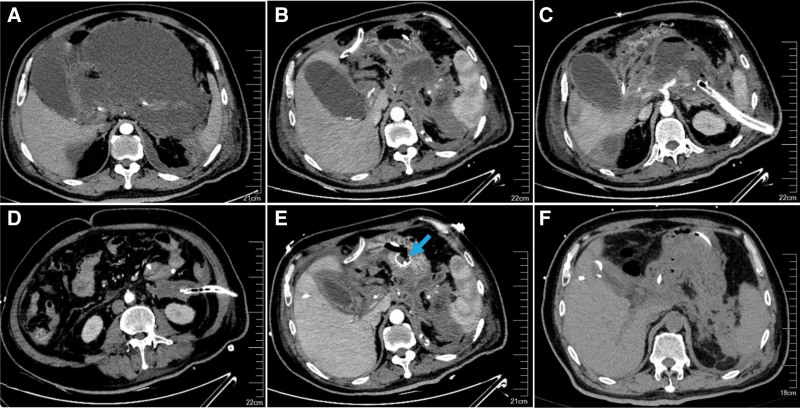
CT images of the abdomen in patient 4. (A) An initial CT scan shows extensive pancreatic necrosis and peripancreatic fluid collection, with a CTSI of 8, (B–D) CT-guided multifunction drainage tubes were placed through previously established percutaneous sinus tract in the perihepatic space, left anterior pararenal space and paracolic gutter at day 14 after PCD were performed. Subsequently, CNPI was initiated through the multifunction drainage tubes, (E) PEN and TN using LAMS was performed simultaneously at day 15 after CNPI were performed. The LAMS was seen in the wall of the stomach (blue arrow), and (F) CT scan was performed at 91 days after the onset of the disease and the images shows that the pancreatic and peripancreatic necrosis was fully removed. CT = computed tomography, CTSI = CT severity index, CNPI = continuous negative pressure irrigation, LAMS = lumen-apposing metal stent, PCD = percutaneous catheter drainage, PEN = percutaneous endoscopic necrosectomy, TN = transgastric necrosectomy.
